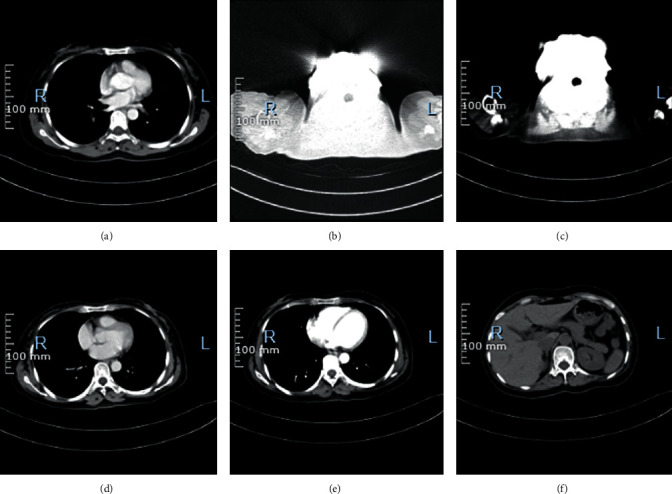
CT images of one patient. (a)–(c) The CT signs of the patient before treatment. Two small cystic shadows without enhancement are observed in the cervix, about 0.8 cm in diameter. Extensive nodular shadows are observed in the peritoneum, uniform enhancement is observed on enhanced scan, and extensive fluid density shadows are observed in abdominal and pelvic cavities. Extensive fluid accumulation in the abdomen and pelvis. (d)–(f) The CT signs of the patient after treatment. The pelvic mass is significantly reduced, and the pelvic effusion is completely absorbed.
